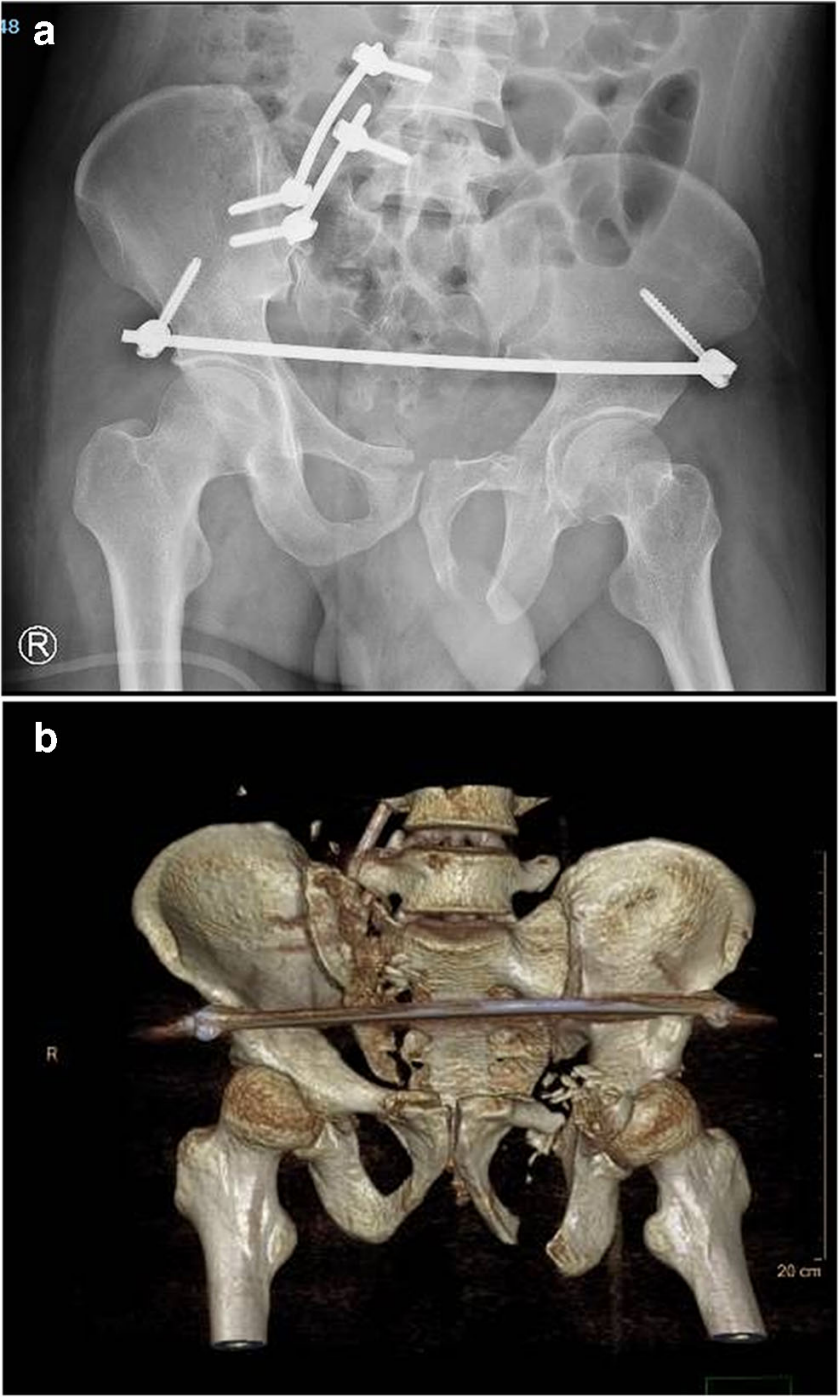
Post-operative images of the unstable pelvic fracture repair. a Post-operative X-ray showing vertical displacement reduction of the right sacroiliac joint at the posterior pelvic ring, reduction and good fixation of the pubic ramus fracture at the anterior pelvic ring. b Post-operative computed tomography (CT) 3D reconstruction showing good pelvic ring reduction and fixation
